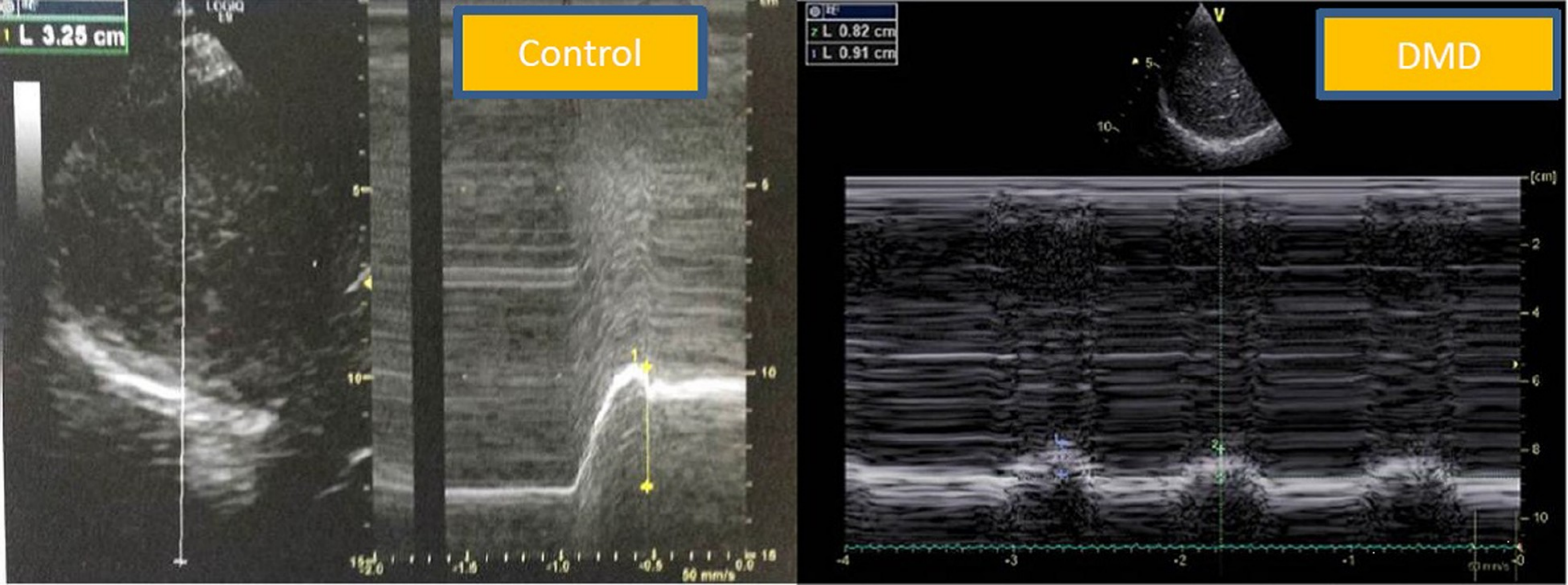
Diaphragm ultrasound displacement from the subcostal view in a control subject and in a DMD patient. Normal right diaphragm sniff time movement (TM) motion in the control group (*left)*; note the sharp upstroke during a sniff maneuver. Pathological right diaphragm motion during a sniff maneuver in a DMD patient (*right*). Note the reduced diaphragm motion with the sniff maneuver. DMD: Duchenne muscular dystrophy.
